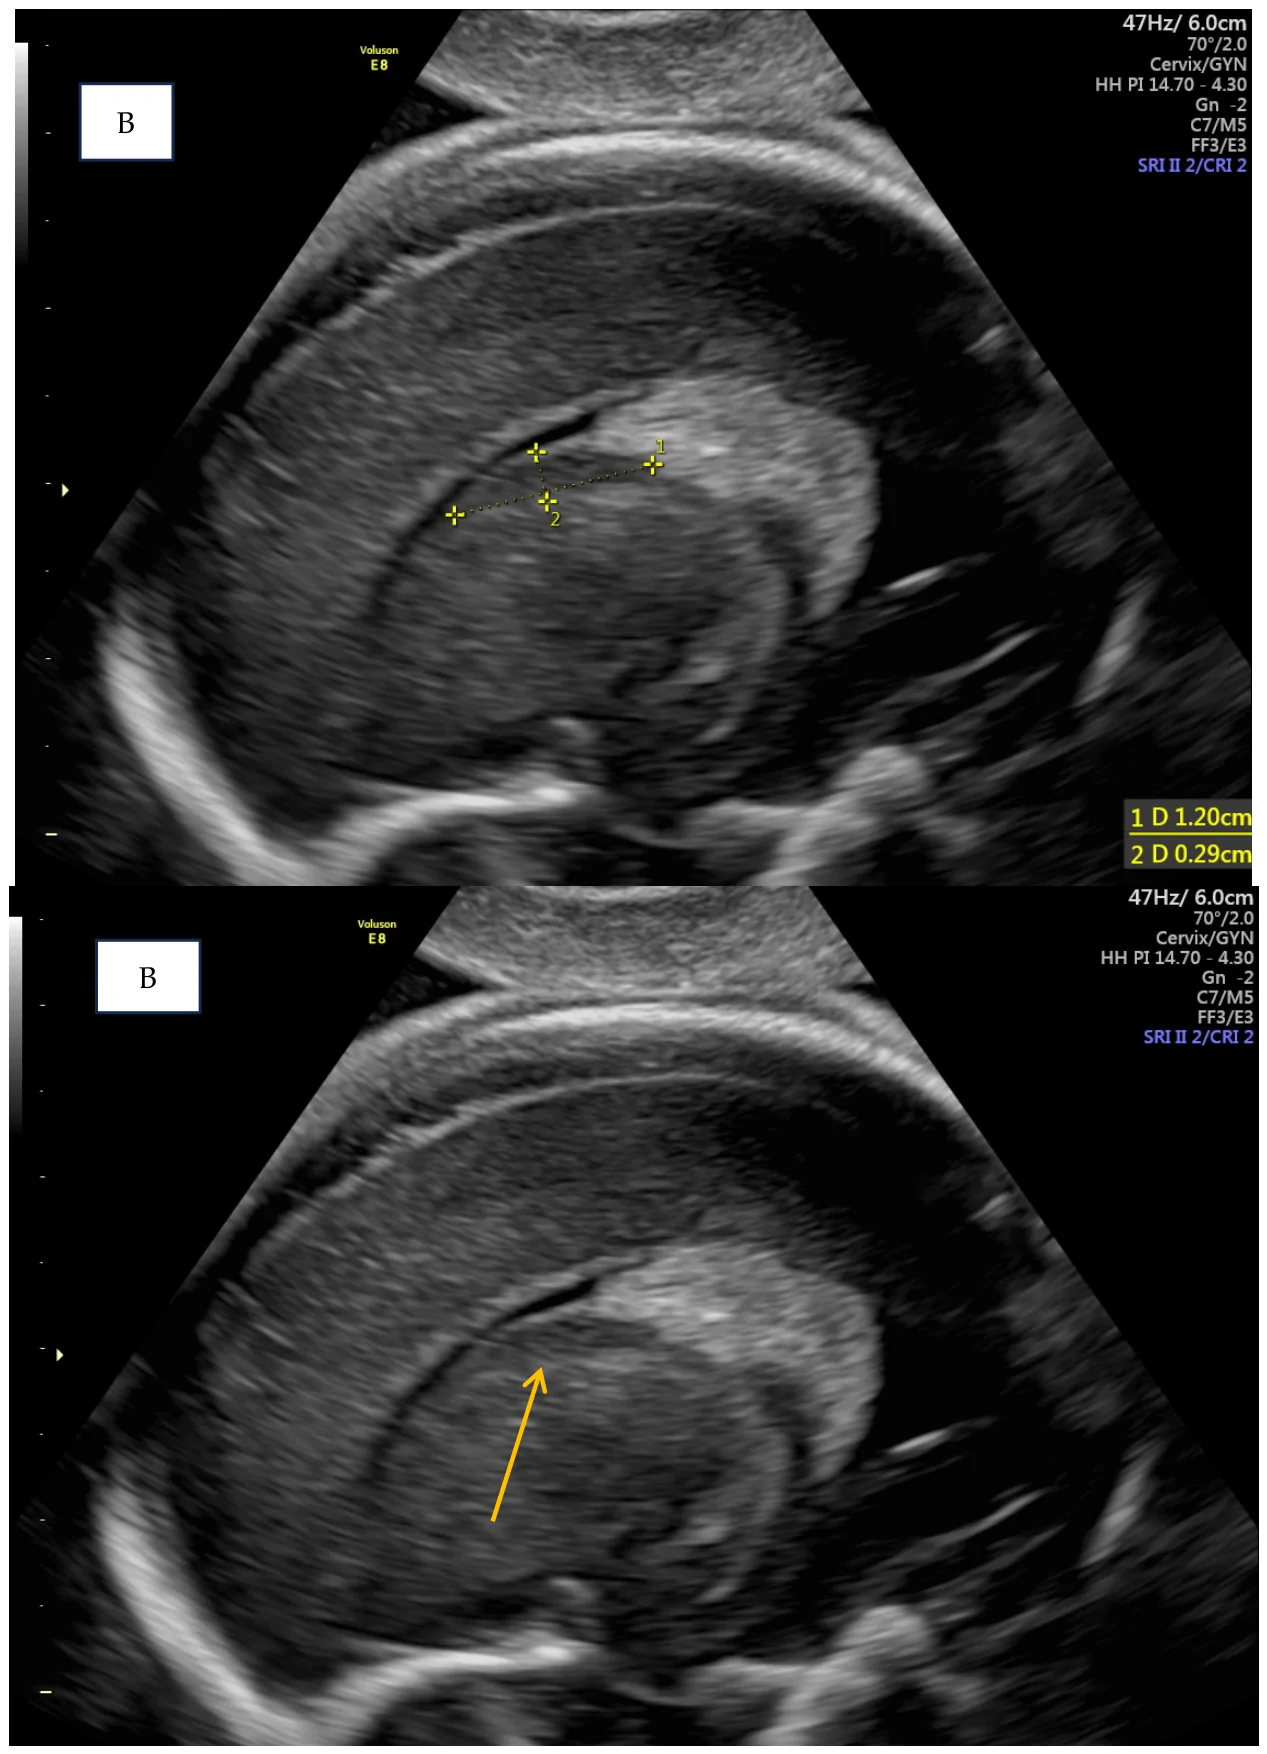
Standard ultrasound planes for the assessment of the fetal ganglionic eminence (GE) using transvaginal neurosonography at 20 weeks of gestation. (A) Coronal view showing the GE (arrow) located inferolateral to the frontal horns of the lateral ventricles. (B) Parasagittal view demonstrating the normal appearance of the GE (arrow) anterior and inferior to the choroid plexus within the caudothalamic groove. This plane is particularly useful for detailed morphological evaluation and measurements of the GE.
